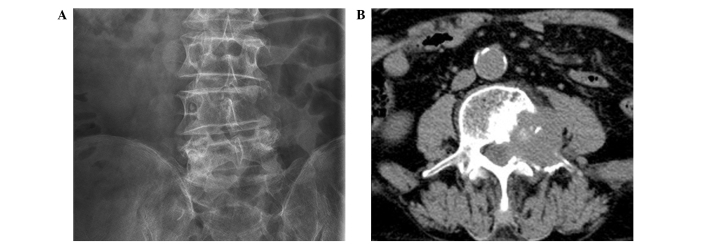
Expansile osteolytic lesion of the L4 vertebra, including erosion of the cortex. (A) A radiograph of the spine revealed an osteolytic lesion of the L4 vertebra involving erosion of the cortex. (B) Computed tomography scans of the lumbar spine revealed the presence of an expansive, lytic and destructive lesion with a cortical attenuation in the L4 vertebra which included a soft tissue component. L4, fourth lumbar.
